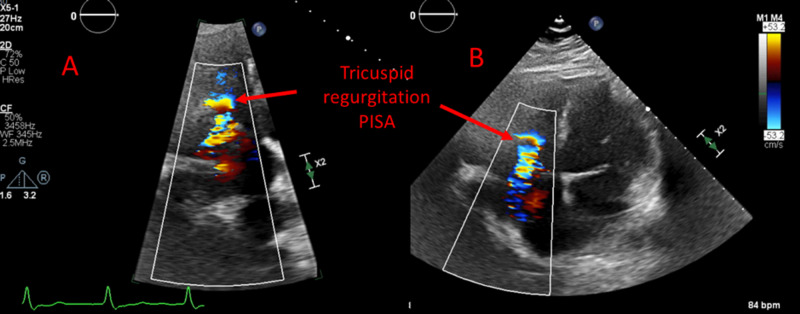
Echocardiography with color doppler: Severe tricuspid valve regurgitation with large Proximal Isovelocity Surface Area (PISA)
